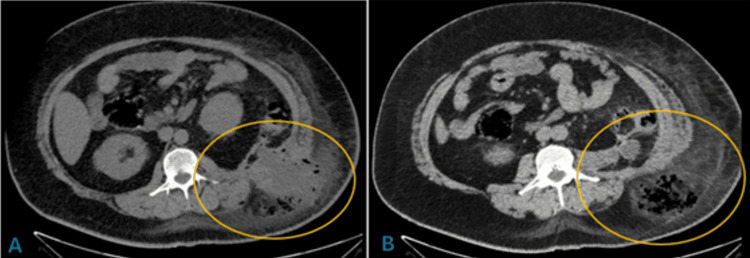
Non-contrast axial abdominal CT scan. (A) A heterogeneous collection at the left flank associated with gas and surrounding fat stranding communicating into the abdominal cavity, involving the colon. (B) Heterogeneous collection is associated with gas and surrounding fat stranding in soft tissues.
